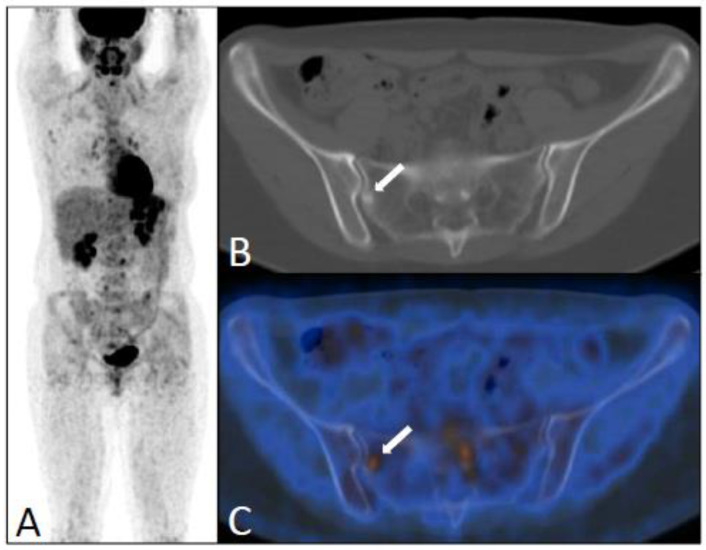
An FDG-PET/CT scan performed five years after the initial diagnosis of ILC (pT3N2, SBR II) of the right breast in a 37-y-old patient because of elevated tumor marker (CA 15.3 = 56) and pain in the thoracic spine. Maximum intensity projection (A) showing locoregional recurrence and bone metastases with low uptake. Axial CT (B) and PET/CT fusion images (C) of the pelvis showing a sclerotic lesion with low increased FDG uptake (white arrows). Hormone therapy was introduced (tamoxifen). This patient died five years later from disease progression with carcinomatous meningitis and uterine metastases.
